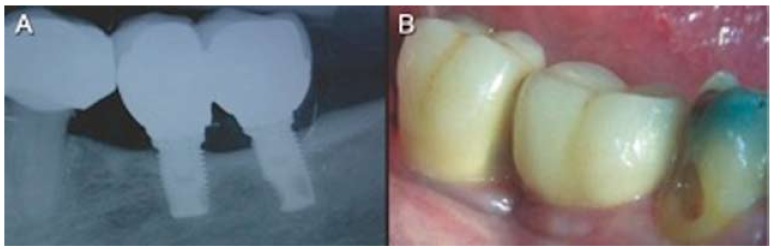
Shorty bridge case. A) Intraoral radiographs of shorty bridge after a mean period of 47.72 months of follow-up. B) Clinical photograph of a shorty bridge.
